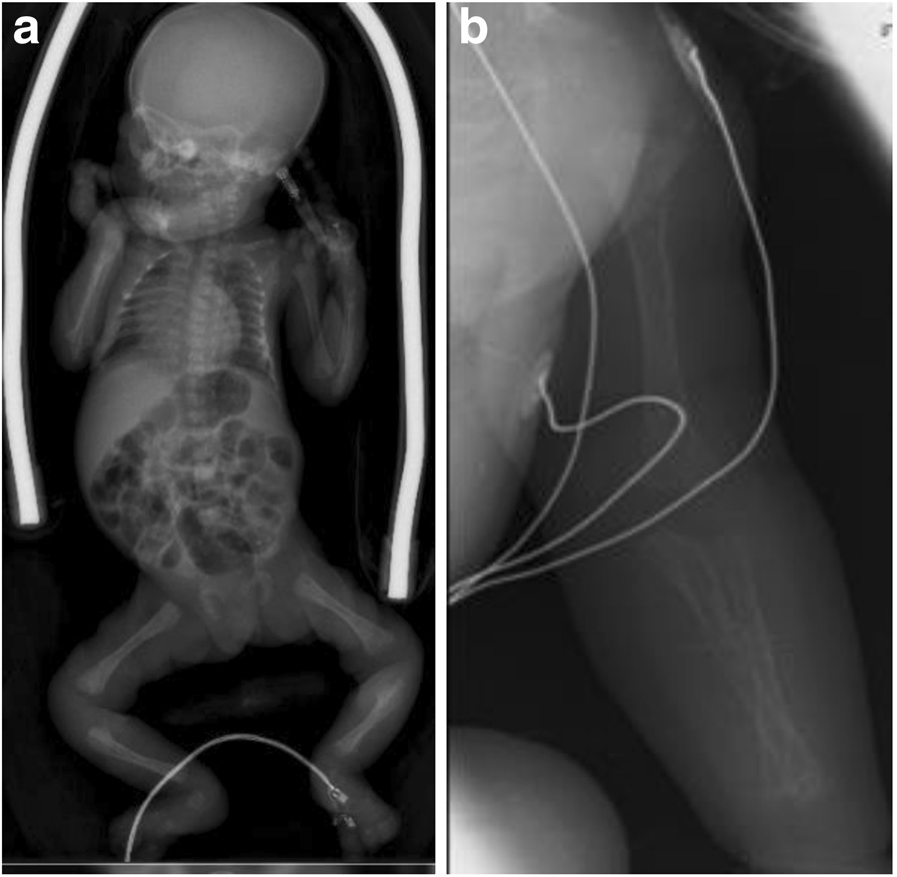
a Radiographic appearance of bone fibrodysplasia. An important cardiomegaly is also observed. b Diaphyseal fracture of the ulna. In McCune–Albright syndrome fractures occur as a result of osteopenia associated with cortical thinning
